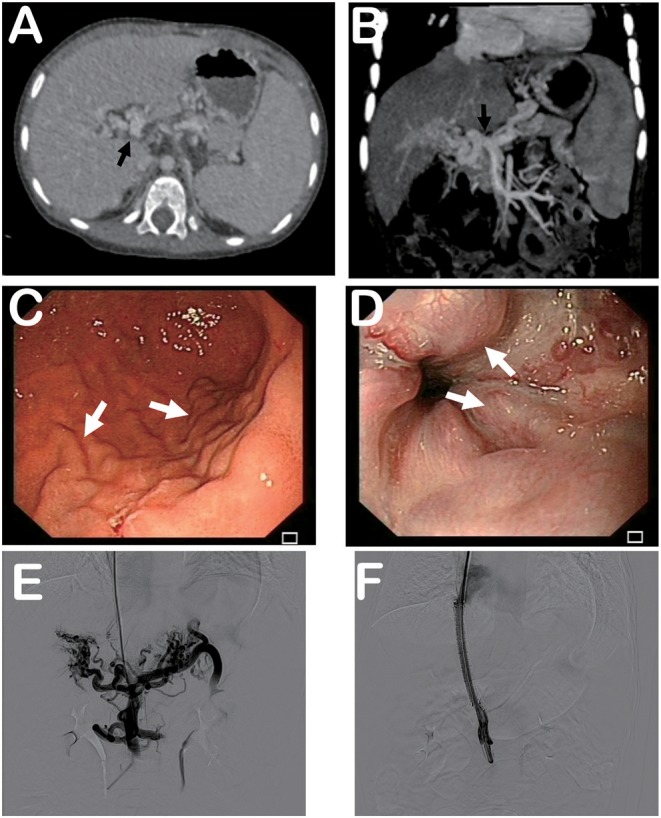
Computed tomography, esophagogastroduodenoscopy, and portography. (A) Computed tomography showing splenomegaly and signs of cavernous transformation of portal vein. (B) Tomographic reconstruction showing cavernous transformation of portal vein. (C) Esophagogastroduodenoscopy demonstrating gastric varices. (D) Esophagogastroduodenoscopy revealing esophageal varices. (E) Portography before stent placement indicating formation of multiple convolute collateral vessels at hepatic hilus. (F) Patency of portal vein and stent was confirmed by portography after stent placement. Black arrows indicate portal vein. White arrows indicate varices.
